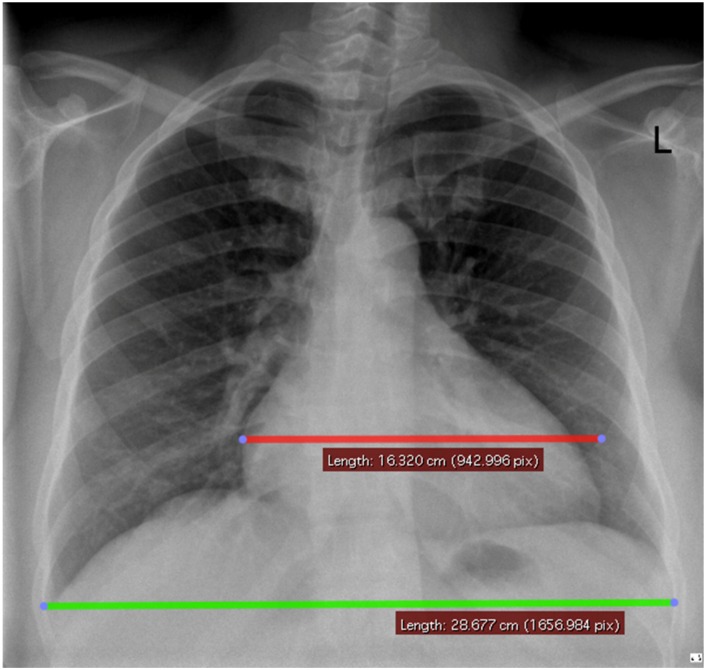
Method for determining Cardiothoracic Ratio. Digital Posterior-Anterior Chest Radiograph (CXR) with maximal cardiac diameter in red and maximal thoracic diameter in green. Cardiothoracic ratio (CTR) is 0.57.
